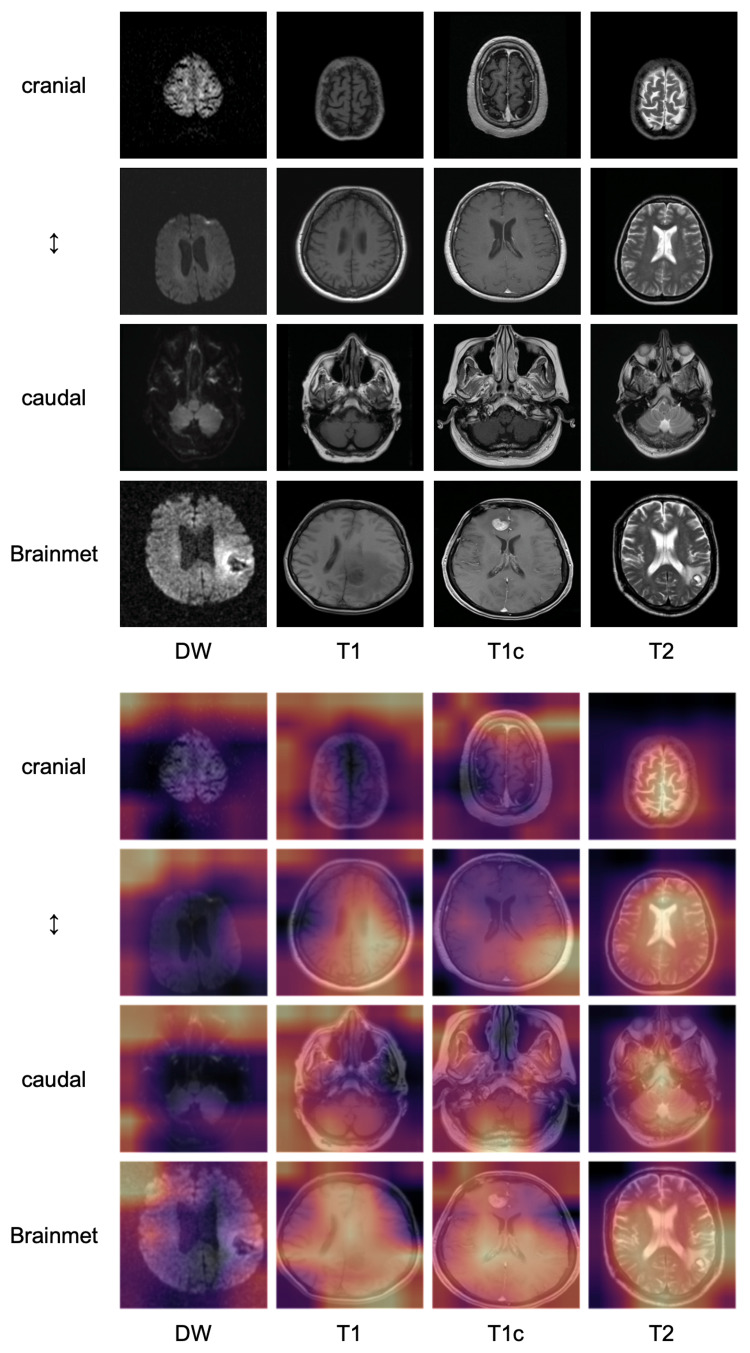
Implementation of Grad-CAM Implementation of Grad-CAM for ResNet-50 on 16 correctly classified images from 16 different patients highlighting the areas of an image the predictions of the model are based on. The top three rows show slices without macroscopic tumors on different levels of the brain, while the bottom row shows slices containing tumors on a similar level. When looking at T2-weighted images, the network tends to highlight areas of the image that contains cerebrospinal fluid. DW, diffusion-weighted; T1, T1-weighted; T1c, T1-weighted with contrast enhancement; T2, T2-weighted
